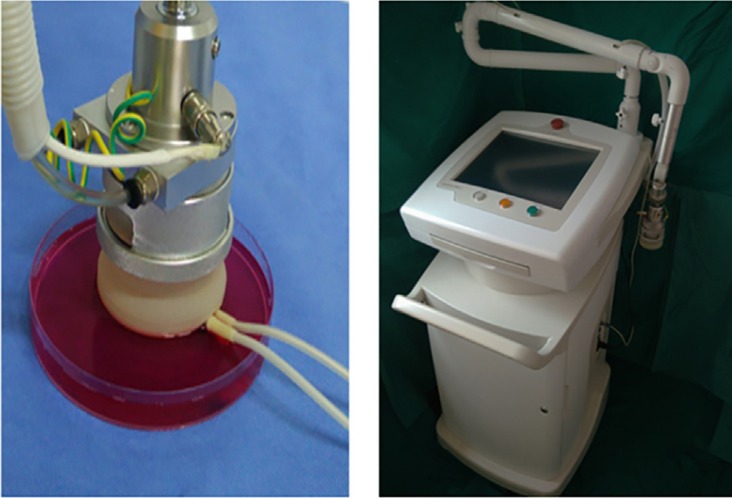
Ultrasonic thermal therapy system.
